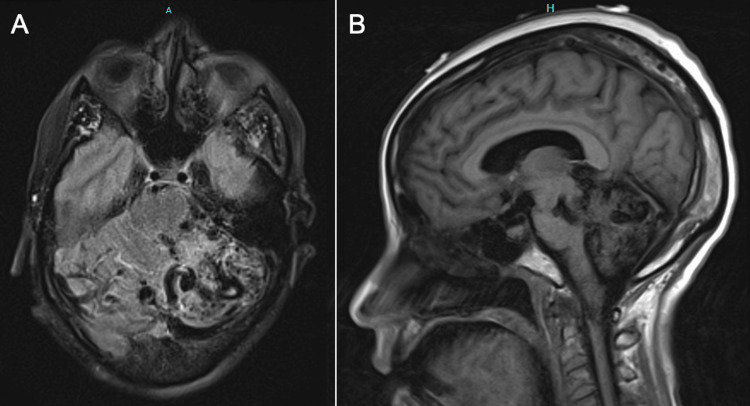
MRI of the brain FLAIR sequence (transverse (A) and sagittal (B) views) highlighting the significant atrophy in the cerebellum and showing a hypodensity in the left thalamus. FLAIR: fluid-attenuated inversion recovery.
